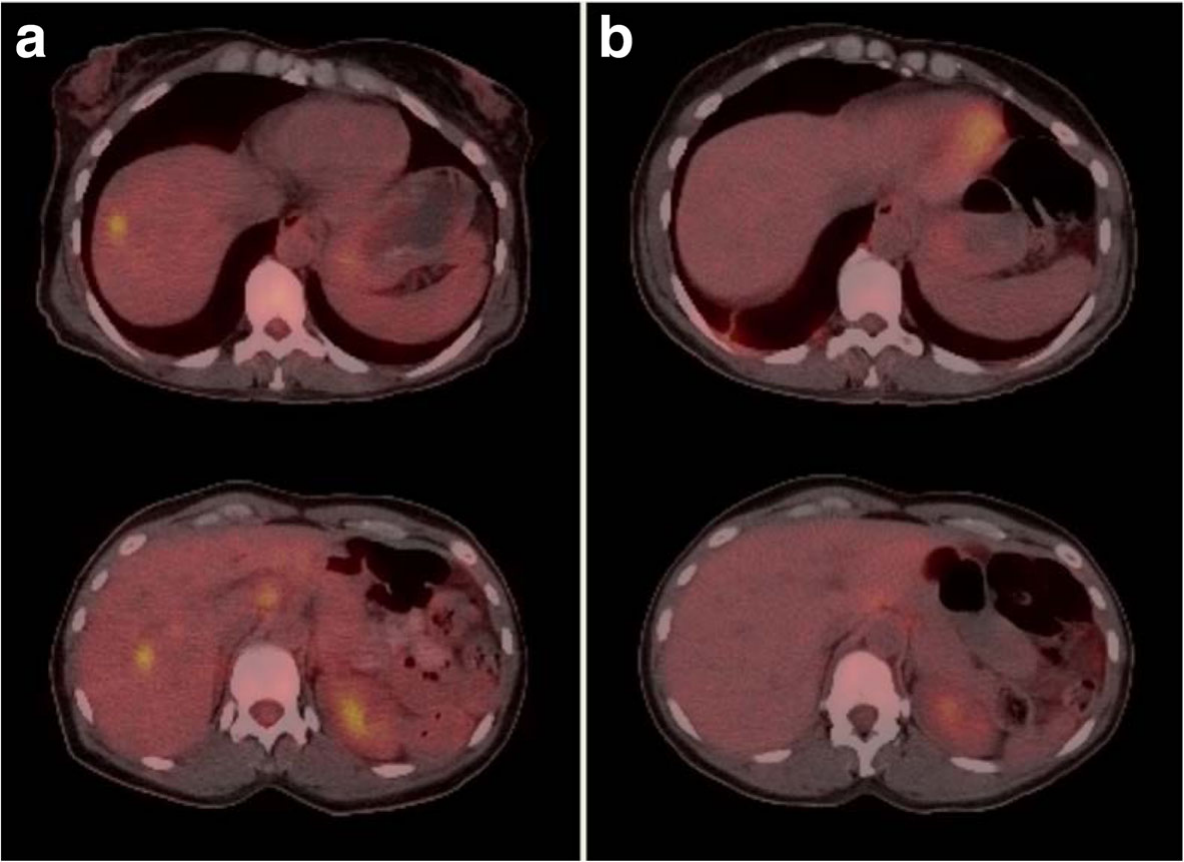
PET-CT of Hepatic Metastases Before and After Combination Immunotherapy. a PET-CT scan at times of diagnosis of with several hypermetabolic hepatic lesions consistent with metastases. b PET-CT scan after 4 cycles of nivolumab plus ipilimumab and 3 cycles of nivolumab maintenance
